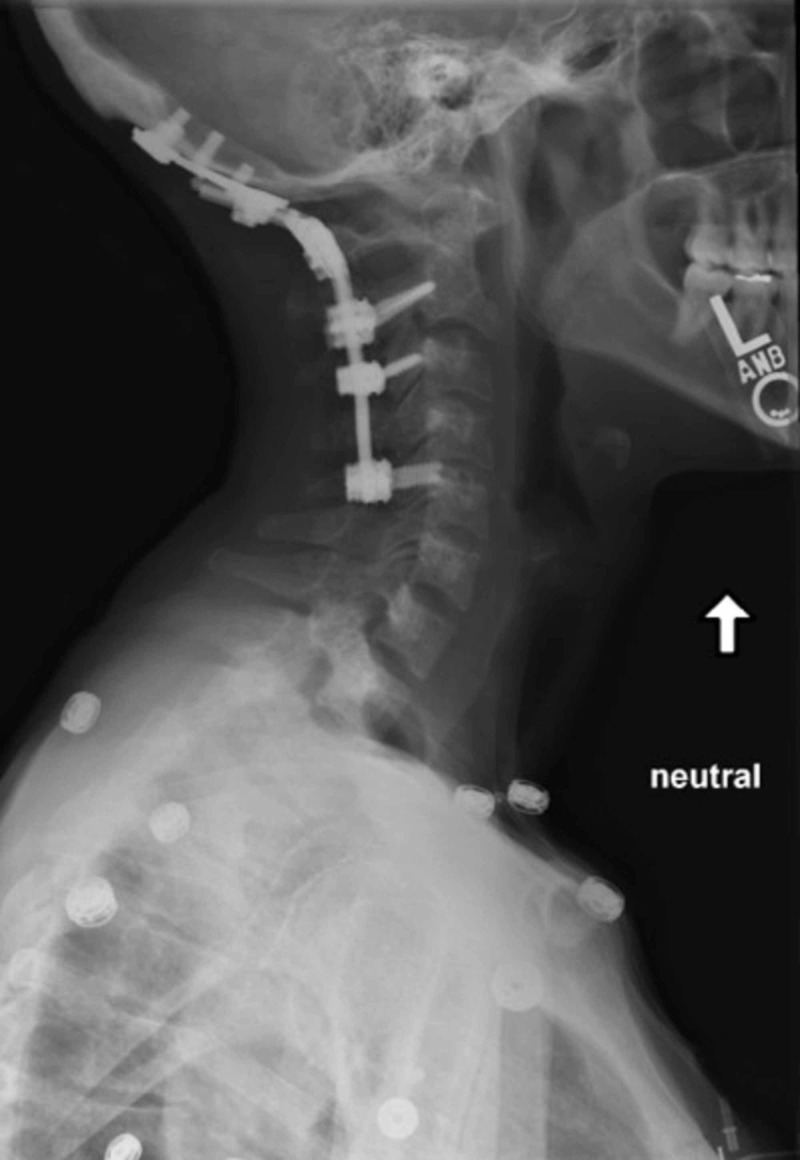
Postoperative lateral X-ray of the cervical spine Postoperative lateral X-ray of the cervical spine obtained at one year showing posterior occipitocervial fusion from the occiput to C5 with intact hardware and restored alignment.
